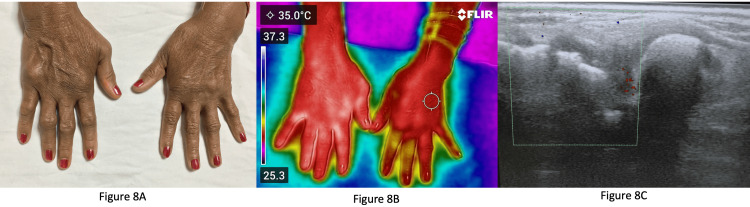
Image of a patient with deforming disease 8A: Clinical image of a patient with deforming disease; 8B: Infrared thermography image indicating increased temperature through all the metacarpophalangeal (MCP) and proximal interphalangeal (PIP) joints; 8C: Ultrasonography image of the right fifth MCP and PIP with synovitis and power doppler uptake.
